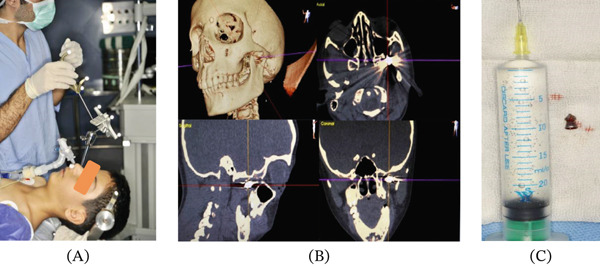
The surgical approach. (A) Reference frame and tool calibration for optical surgical navigation. (B) Intraoperative 3D CT scan with different views (axial, sagittal, and coronal) of FB location. (C) The extracted FB. CT, computed tomography; 3D, three‐dimensional; FB, foreign body.
